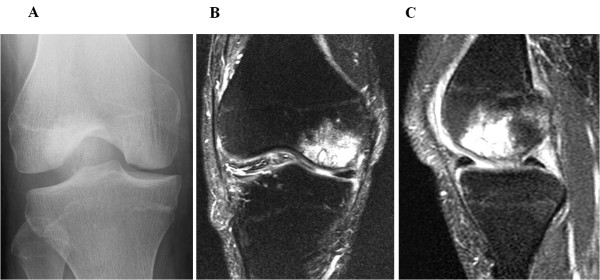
Preoperative findings in case 3. (A) Anteroposterior radiograph reveals an osteonecrotic lesion in the right medial femoral condyle. (B,C) Coronal and saggital MRI shows an osteonecrotic lesion with high signal change on the T2-weighted fat suppression image in the right medial femoral condyle.
